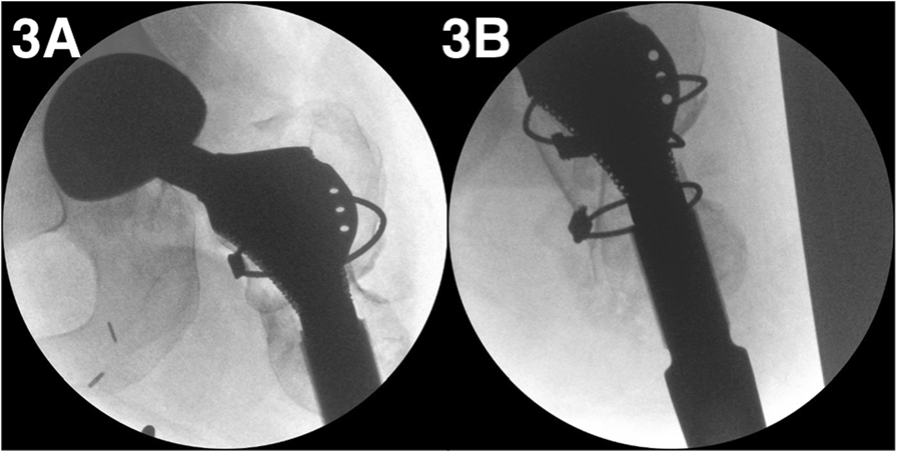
Examples of intraoperative radiographs for case 3, 3A antero-posterior view, 3B lateral view
